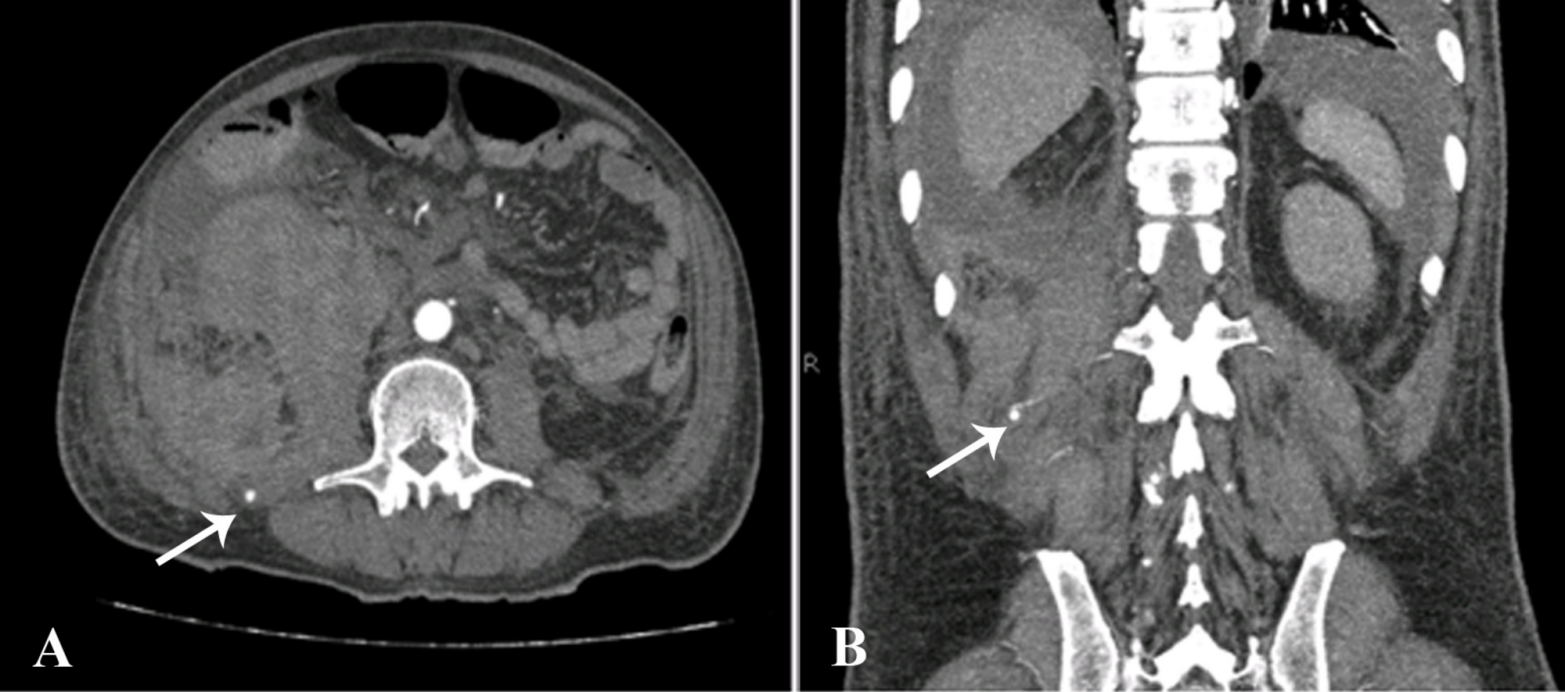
CT abdomen and pelvis angiogram Axial (A) and coronal (B) images from the arterial phase showing a small saccular pseudoaneurysm arising from the right L2 lumbar artery (arrows). CT: computed tomography
